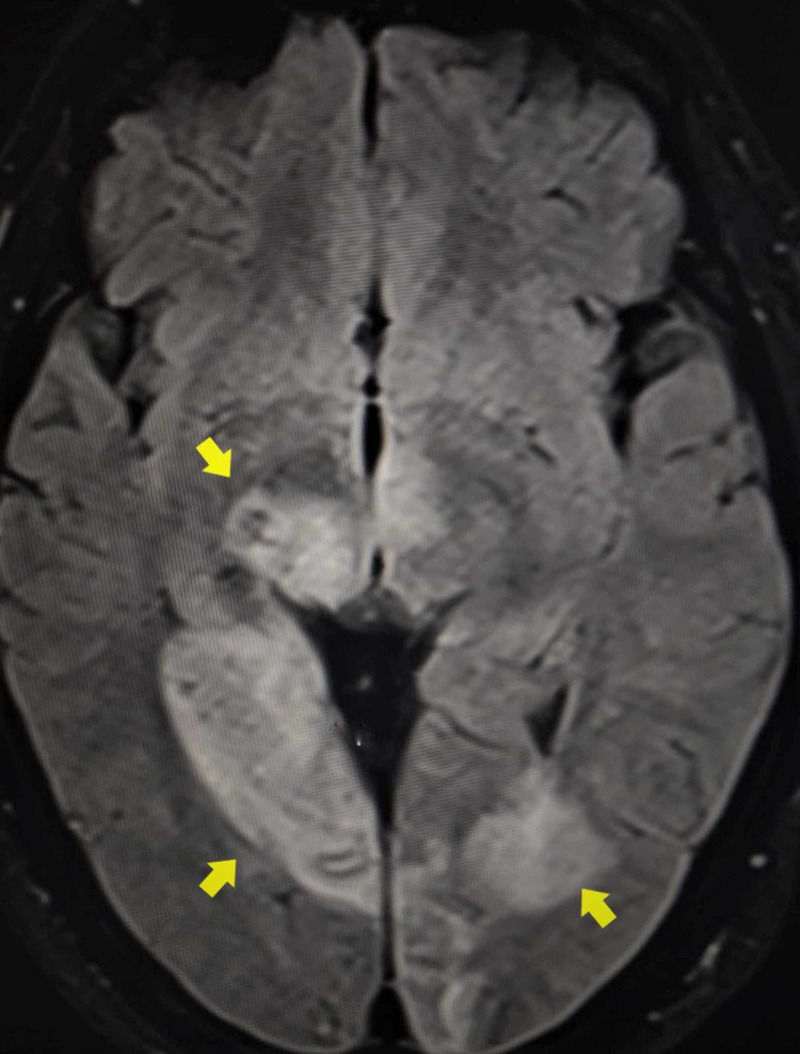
FLAIR sequence of MRI The image shows areas of hyperintensities in the bilateral occipito-parietal-temporal regions and bilateral thalamus (yellow arrows) MRI: magnetic resonance imaging; FLAIR: fluid-attenuated inversion recovery
